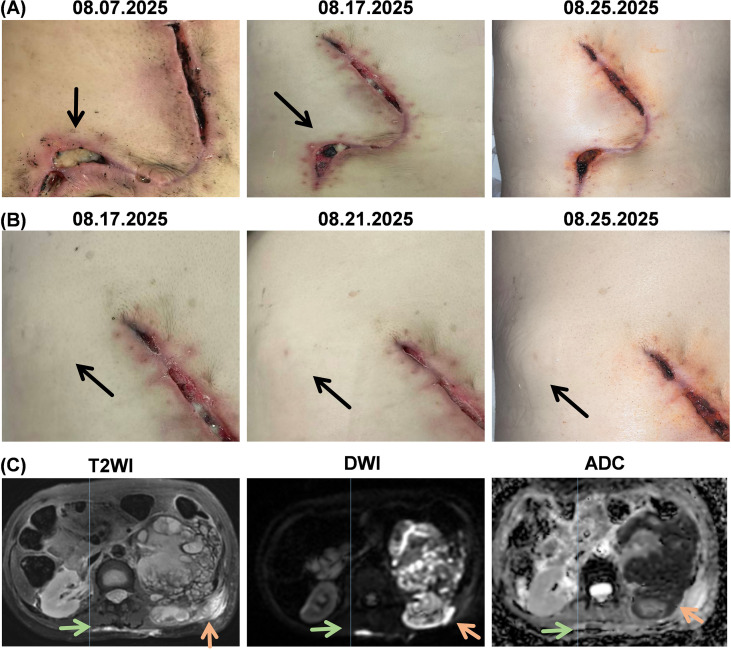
Back and nephritic lesions during third hospitalization. (A) 2025.08.07: Back incision dehiscence with persistent abscess-like fluid drainage. From 2025.08.17 to 2025.08.25, no significant improvement in incision condition following dressing changes; (B) 2025.08.17: First detection of a subcutaneous abscess at the 10 o’clock position of the back incision. The subcutaneous abscess gradually enlarged between 2025.08.21 and 2025.08.25; (C) 2025.08.24 MRI findings for aseptic inflammatory abscesses of the back and nephritic abscess: Low signal intensity on T2-weighted images (T2W1) within the cyst wall, diffusion suppression on diffusion-weighted imaging (DWI), and corresponding apparent diffusion coefficient (ADC) mapping.
